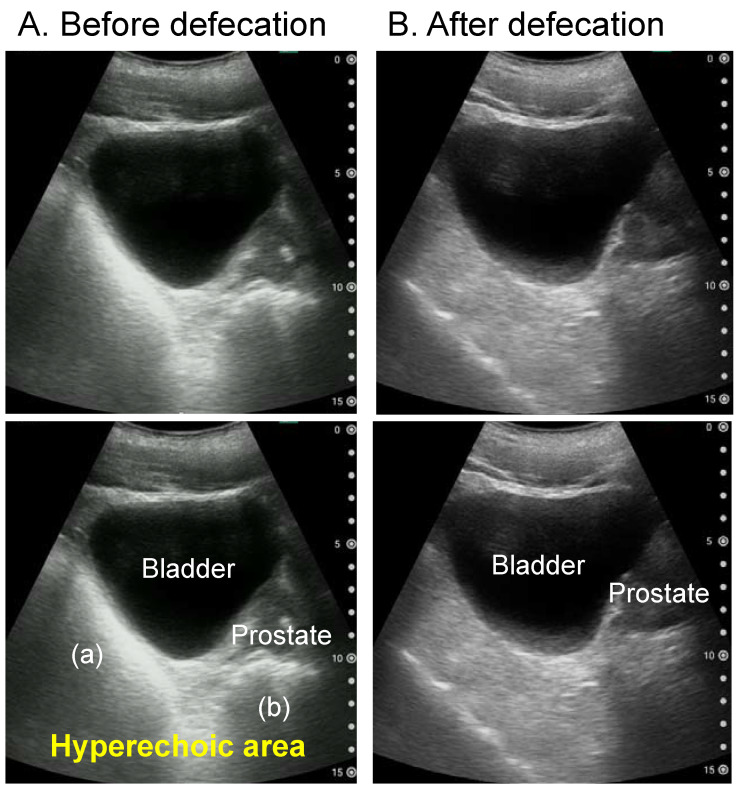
Longitudinal rectal ultrasound images of a healthy male in his 30s before (A) and after (B) defaecation. The top two images are the original ultrasound images, whereas the respective bottom images describe the findings. Before defaecation, there was faecal retention in both the upper (a) and lower (b) rectum. There is no faecal retention in rectum.
